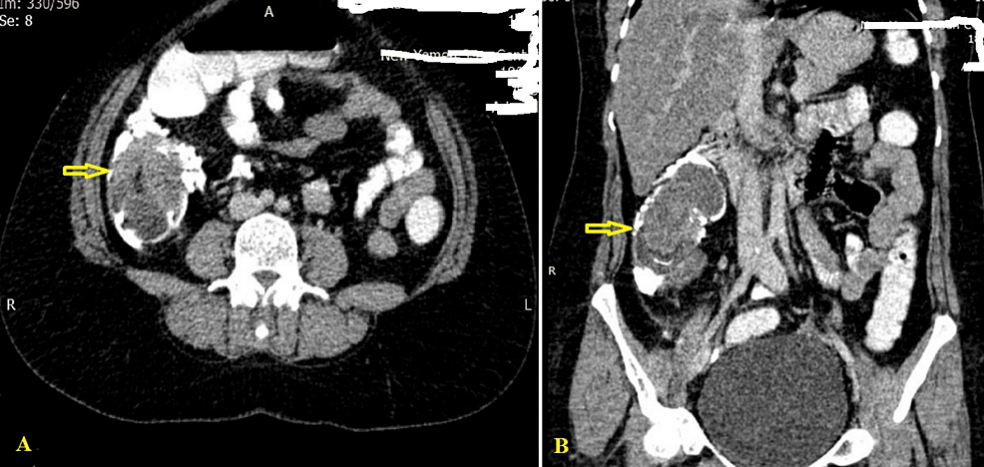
Abdominal/pelvic CT scan with intravenous, oral, and rectal contrast showing the intramural hematoma circumferential wall thickening with luminal stenosis at the ascending colon (arrow). (A) Axial view and (B) coronal view
